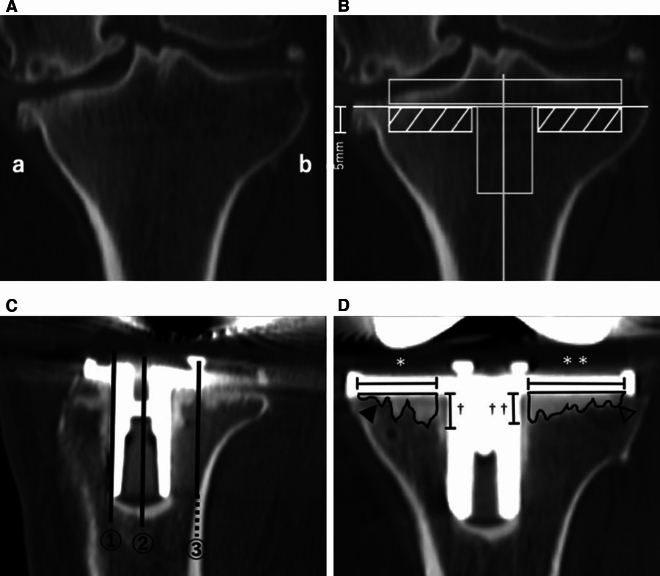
Evaluation method of pre- and postoperative Hounsfield unit (HU) values and cement penetration in computed tomography (CT) images. A Preoperative CT coronal section. (a) Sclerotic side and (b) nonsclerotic side. B Preoperative CT coronal section. Vertical and horizontal lines represent the tibial axis and the osteotomy line in operation, respectively. The shaded area is the estimated area of bone penetration by cement and was defined as the length of the base plate multiplied by 5 mm (the average HU values in the area are calculated by the imaging software). C Postoperative CT sagittal section. (1) Anterior edge of the keel, (2) center of the keel, and (3) extension of the posterior cortex. D Postoperative CT coronal section. The solid arrowhead indicates the cement penetration area of the sclerotic side (mm^2^), The open arrowhead indicates the cement penetration area of the nonsclerotic side (mm^2^). The single asterisk indicates the base of the sclerotic side (mm). The double asterisk indicates the base of the nonsclerotic side (mm). The single dagger presents the maximum penetration in the sclerotic side (mm). The double dagger refers to the maximum penetration in the nonsclerotic side (mm). Mean penetration: area/base (mm)
